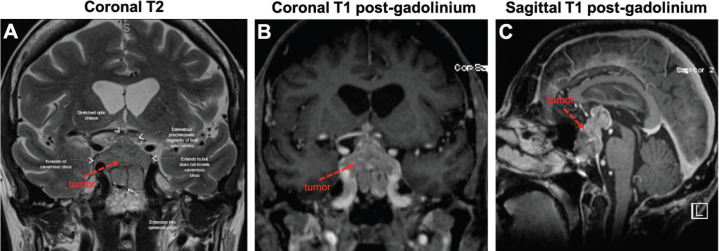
Large sellar/suprasellar mass on MRI. Views of the tumour (red arrows) on coronal T2 (A), coronal T1 with gadolinium (B) and sagittal T1 with gadolinium (C). As pointed out in (A), the tumour invades the right cavernous sinus and extends into the sphenoid sinus. The tumour also compresses the optic chiasm and both optic nerves appear oedematous.
